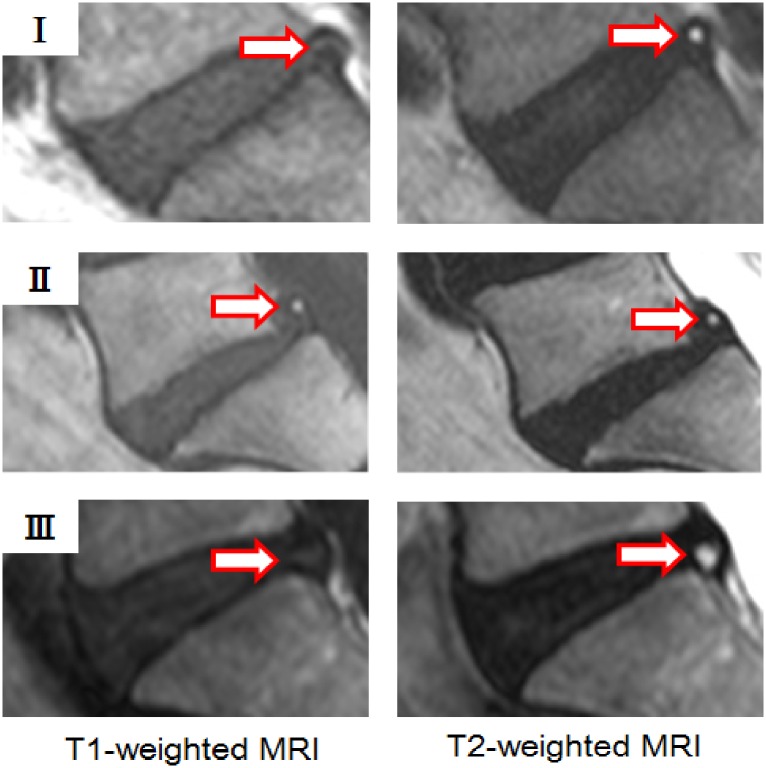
High Intensity Zones based on signal types on T1- and T2- weighted MRI. Three types of High Intensity Zones (HIZ) were created based on the signal type on T1-weighted MRI (low-intensity, high-intensity, and iso-intensity signal) and T2-weighted MRI (high-intensity signal). (I) T1-weighted low-intensity and T2-weighted high-intensity image, (II) T1-weighted high-intensity and T2-weighted high-intensity image, and (III) T1-weighted iso-intensity and T2-weighted high-intensity.
